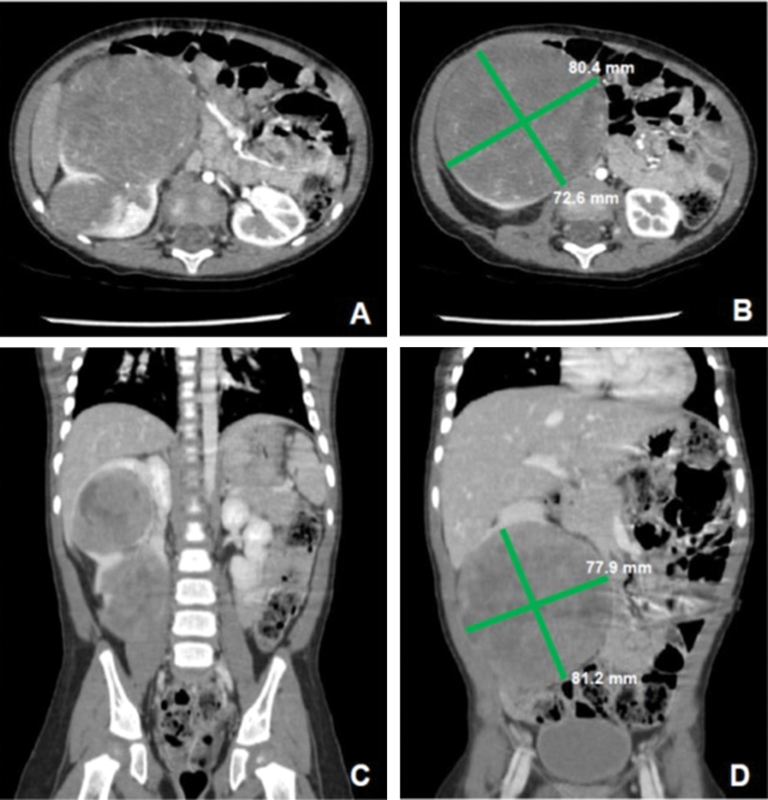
Abdominal computed tomography scan with intravenous contrast. (A, B) (Superior to inferior cuts) axial view; (C, D) (posterior to anterior cuts) coronal view; 8 × 7 cm right kidney tumor.
